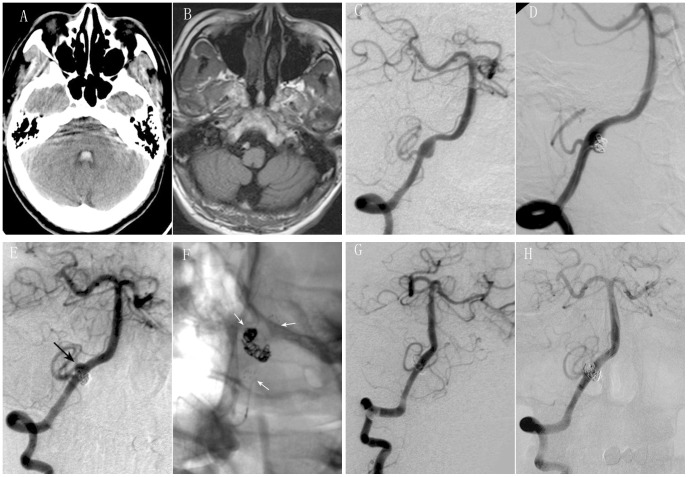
Retreatment with a second stent assisted coiling was performed for recurrent intracranial VADA after SAC. A 43-year old male presented with severe headache. CT scan showed subarachnoid hemorrhage and intraventricular hemorrhage (A). MR imaging showed intramural hematoma (B). Right vertebral angiograms of oblique view showed a dissecting aneurysm involving PICA (C). SAC were performed with near complete occlusion (D). Follow up angiography after eight months revealed regrowth of dissecting aneurysm on the opposite side and in a different location from the original dissecting aneurysm (black arrow) (E). Retreatment by SAC (white arrows) (F) was performed with complete occlusion (G). Follow up angiography after ten months of retreatment showed not only complete occlusion of dissecting aneurysm, but also patency of parent artery (H).
